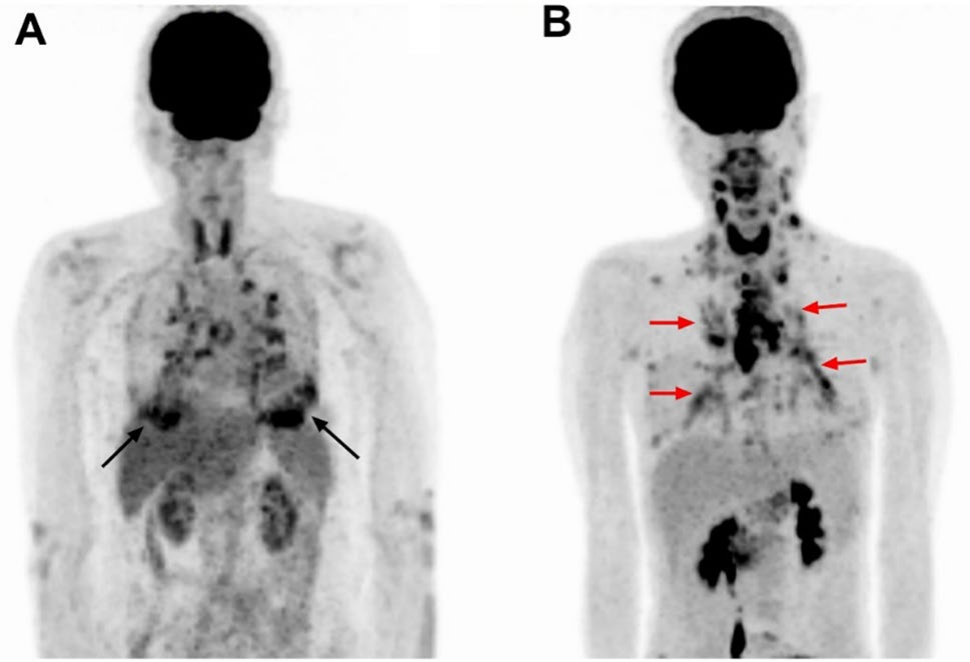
A, Maximum intensity projection 18F-FDG PET/CT image in a 63-year-old breast cancer woman with pulmonary sarcoidosis showed intense tracer uptake in the peripheral area of both lungs (black arrows). B, Maximum intensity projection 18F-FDG PET/CT image in a 50-year-old colon cancer woman with pulmonary lymphangitic carcinomatosis showed intense tracer uptake in the central area of both lungs (black arrows)
